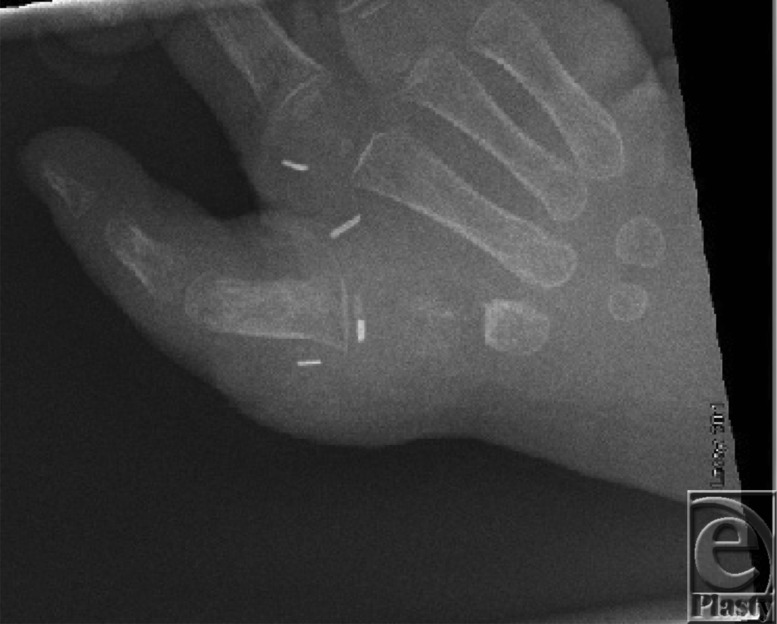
Radiograph of N.D. postoperatively, demonstrating the pollicized index finger.
